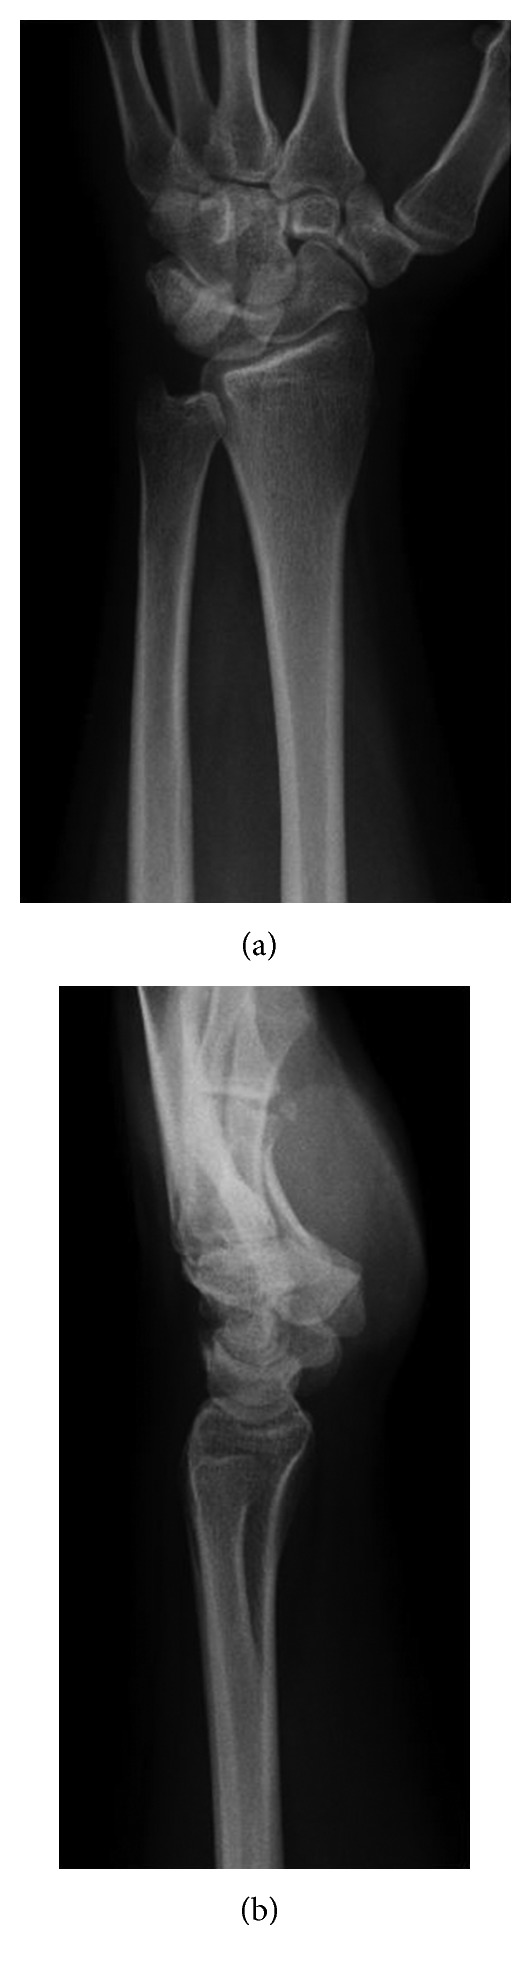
Radiographs at 18 months postoperatively. (a) Posterior-anterior view and (b) lateral view.
